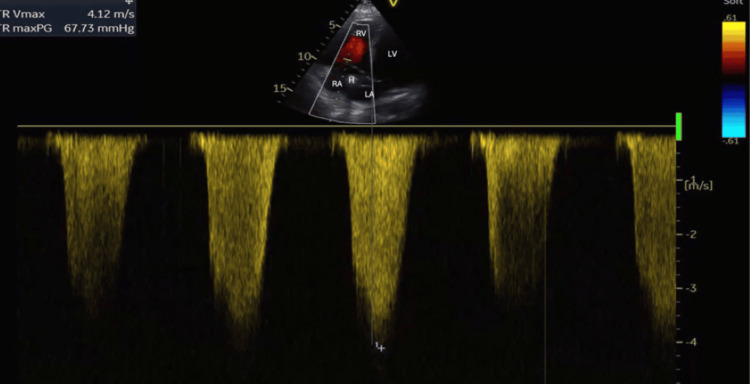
Estimation of pulmonary artery systolic pressure (PASP) assessed by point-of-care ultrasound of the heart. Probe: Phased array. View: Apical four-chamber view using continuous wave Doppler across the tricuspid valve. Depth: 17 cm. RA, right atrium; RV, right ventricle; LA, left atrium; LV, left ventricle; H, HeRO graft tip; TR Vmax, tricuspid regurgitation maximal velocity; TR maxPG, tricuspid regurgitation maximum pressure gradient
